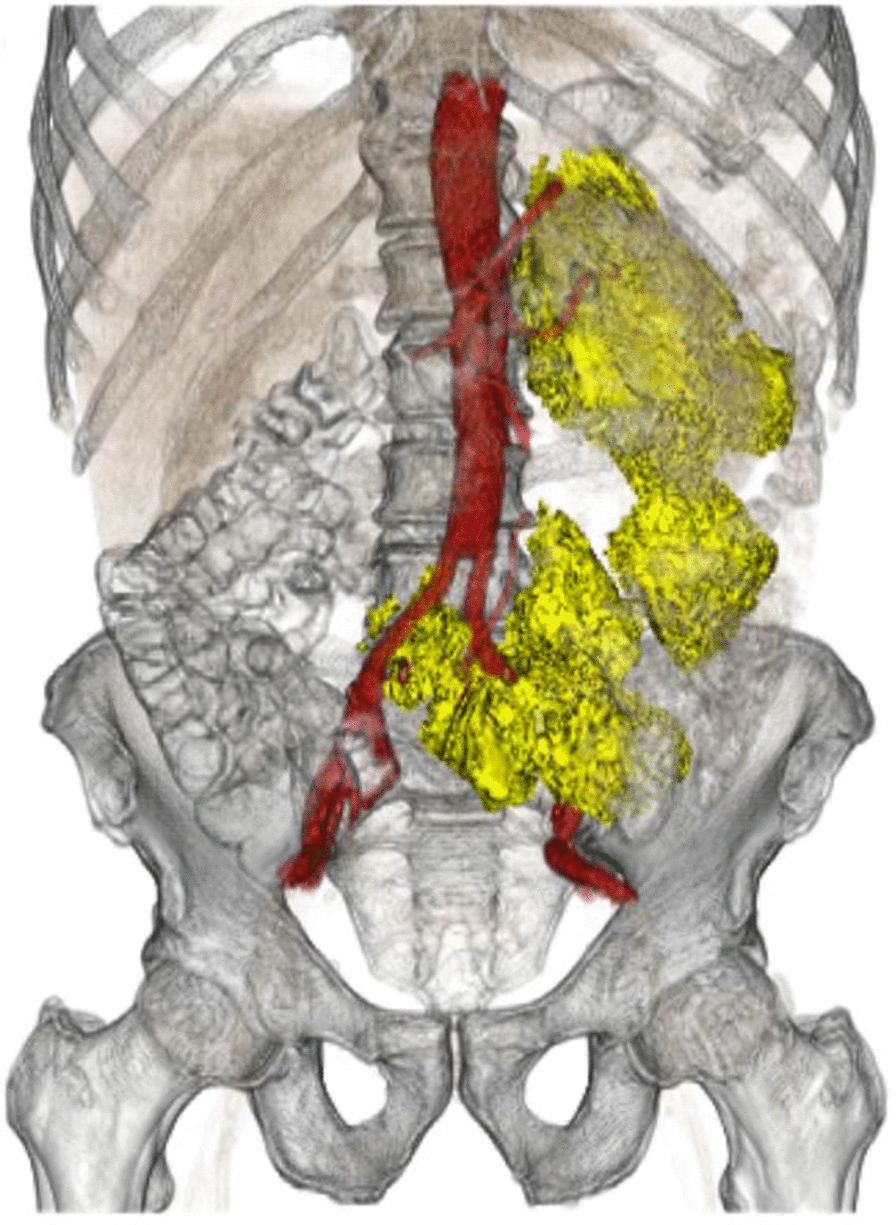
CT- reconstruction showing massive retroperitoneal fluid mass affecting left branch of aortobiiliac graft
